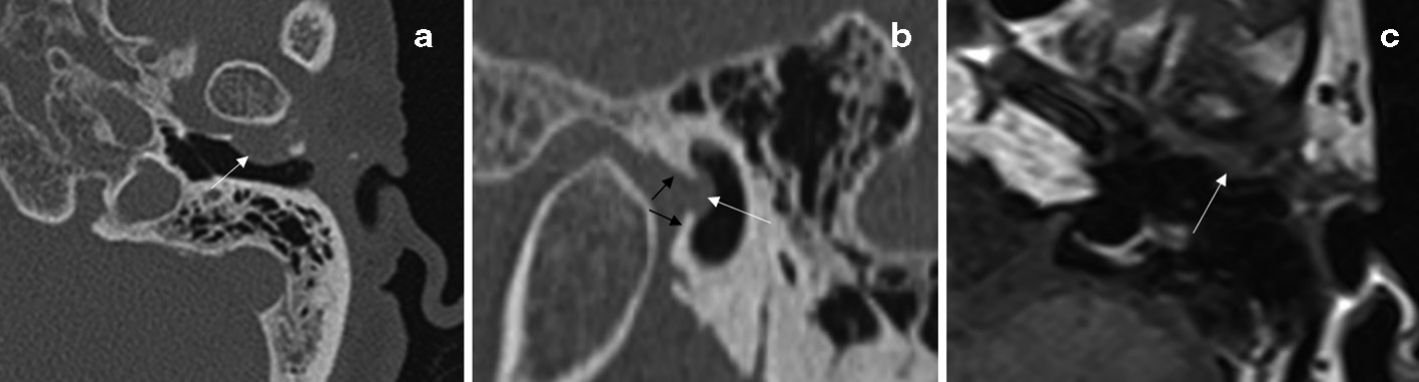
Images of STMJH (from the same patient). (a and b) The CT image, the image (a) showed a thin bony shell around the soft mass; the image (b) displayed the sign of “holding a ball” (black arrows). (c) The MR image, T1-weighted MR image in the axial plane, showed the bulging soft tissue protruded from the TMJ into the EAC
